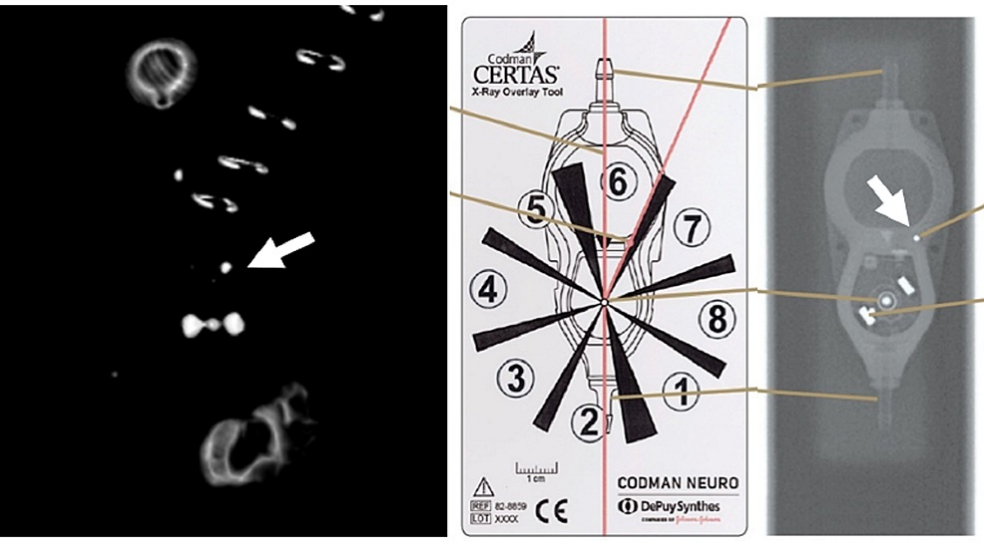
CertasTM Plus volume rendered image reconstruction from routine non-contrast head CT The shunt setting is predicted compared with the manufacturing guide and was correctly predicted as four. The lobes that come closest to the center marker is the one with the extra metal dot, assisting in reading the setting correctly. The setting is compared with the manufacture guidelines in relation to the radiopaque marker (arrow).
